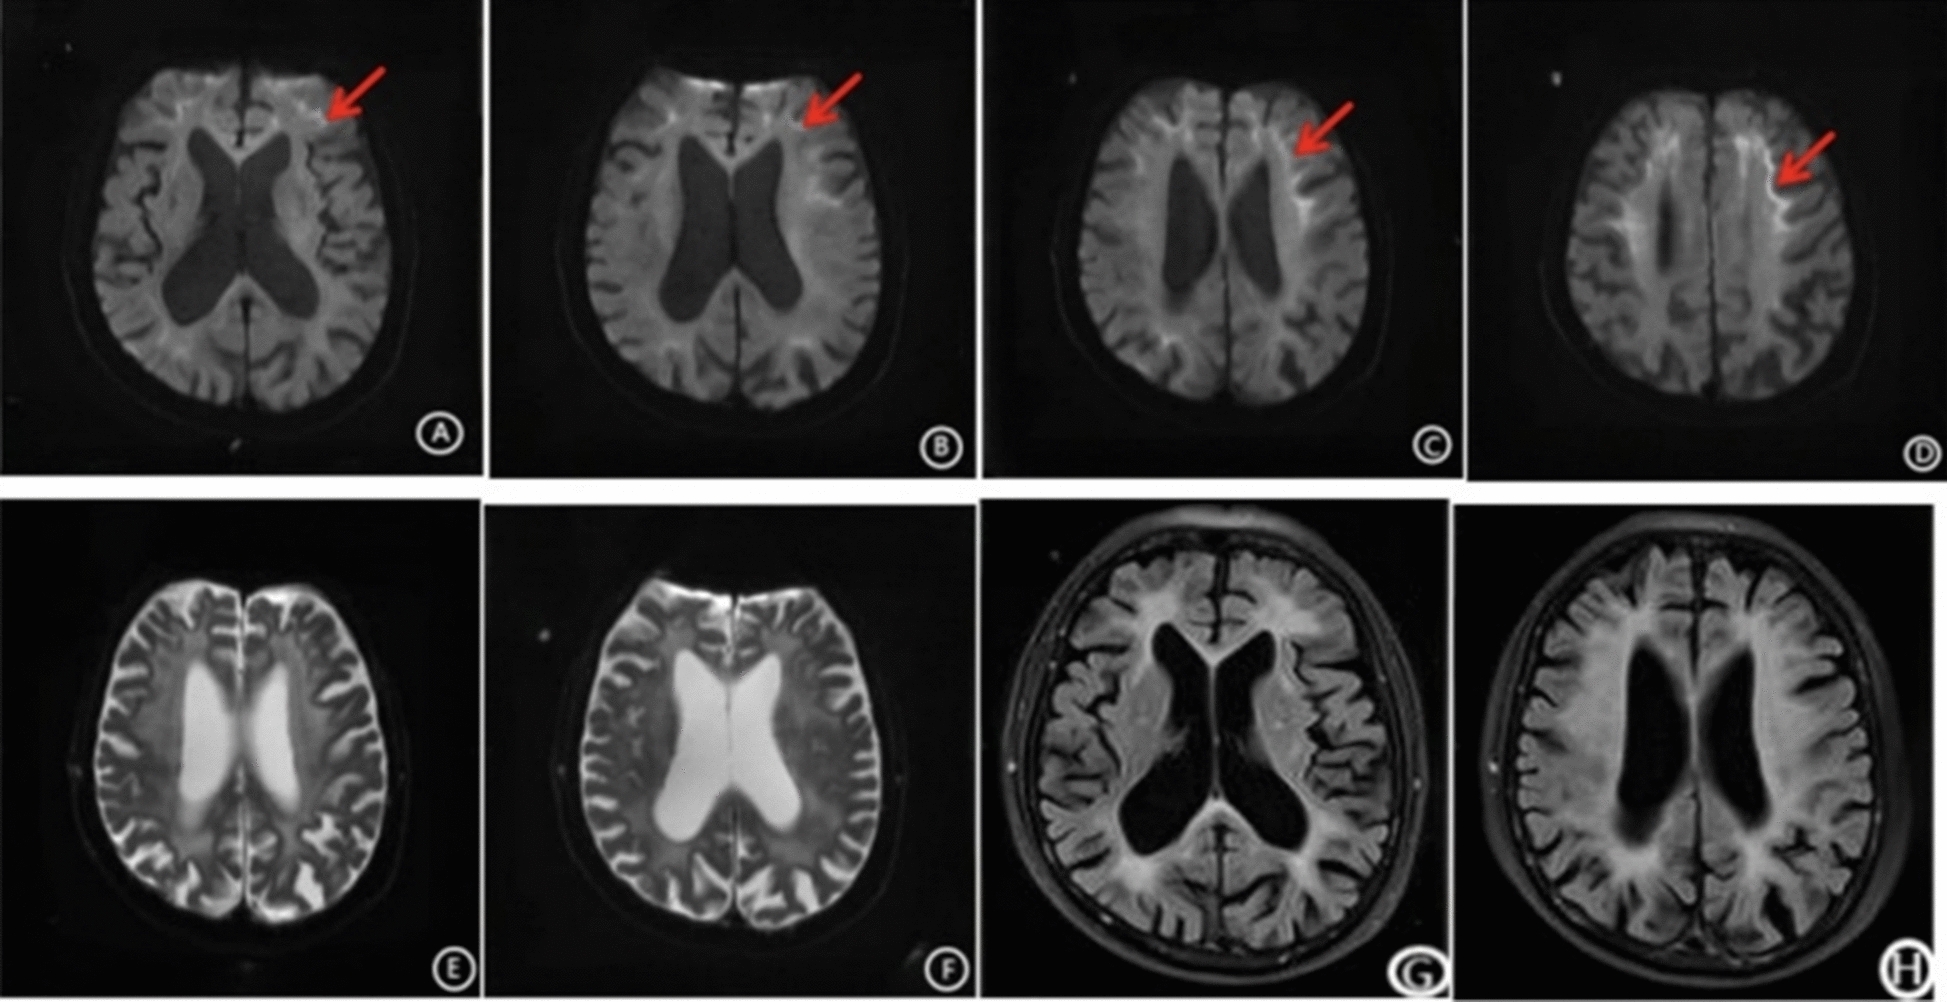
The patient's MRI scanning revealed the following findings: A-D: DWI showed bilateral “ribbon-like” hyperintensities at the corticomedullary junction of the frontal and parietal lobes, resembling the “lace sign”(arrows); E, F: T2-weighted imaging demonstrated bilateral periventricular white matter hyperintensities; G, H: T2 FLAIR sequences revealed diffuse confluent white matter changes
